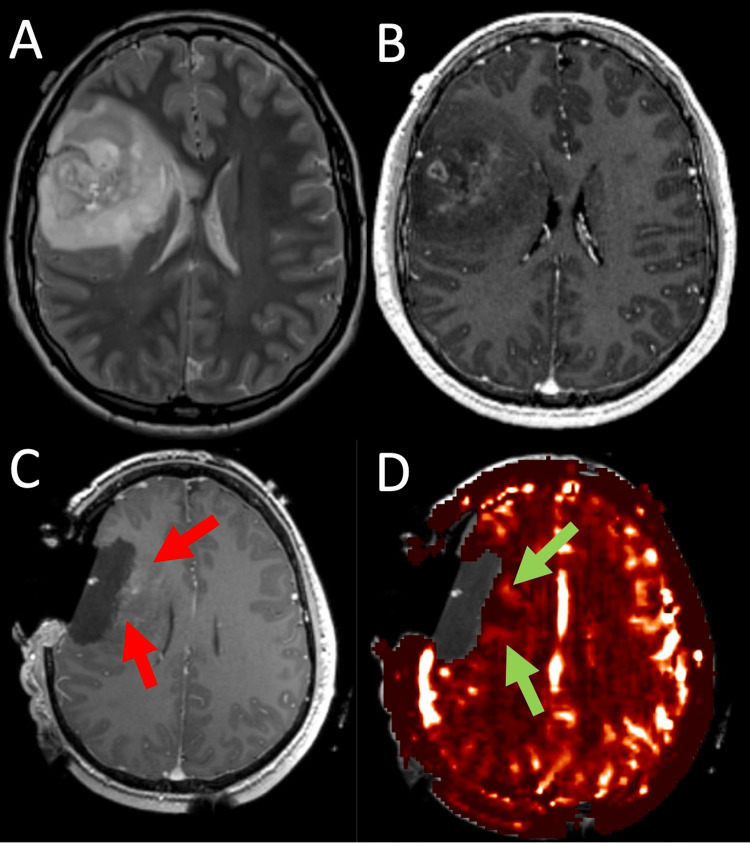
Preoperative MRI, Intraoperative MRI, and Intraoperative Perfusion of Anaplastic Oligodendroglioma A) Preoperative axial T2 and B) T1 contrast-enhanced imaging demonstrate a right frontal anaplastic oligodendroglioma with mass effect and some patchy enhancement. C) Intraoperative T1 contrast-enhanced imaging shows a resection cavity with some faint perilesional enhancement (red arrows). D) Axial dynamic contrast-enhanced perfusion with quantification of Vp overlayed on a T1 contrast-enhanced image shows some faint increased plasma volume (green arrows).
